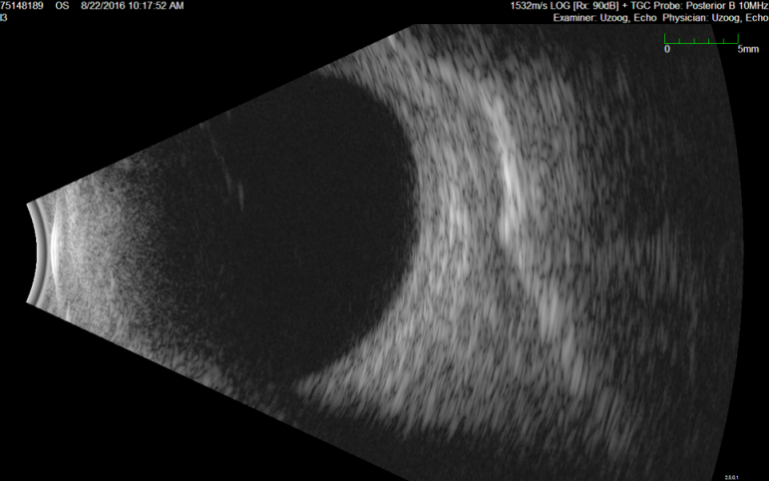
B-scan ultrasonography revealed no fluid in Tenon’s capsule, excluding posterior scleritis.
